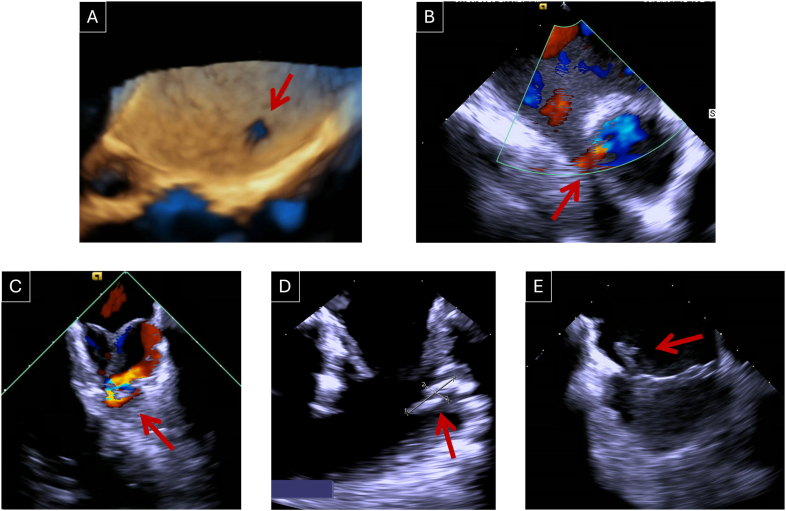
Additional findings indicated by red arrows: A- 3D view of an ostium secundum ASD. B- Color Doppler image showing unroofed coronary sinus with right to left shunt. C- Color Doppler image showing the aortopulmonary fistula. D- 2D image showing a thrombus in the MPA. E − 2D image of a thrombus on the pacemaker lead. ASD, atrial septal defect; MPA, main pulmonary artery.
